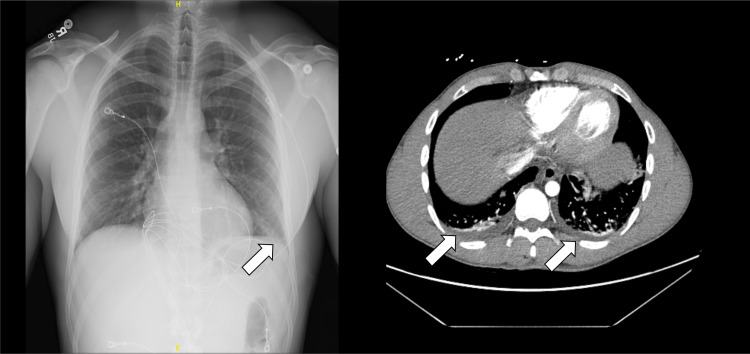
Initial chest radiograph and computed tomography angiography (CTA) of the chest demonstrating a subtle lower lobe infiltrates (arrows).
